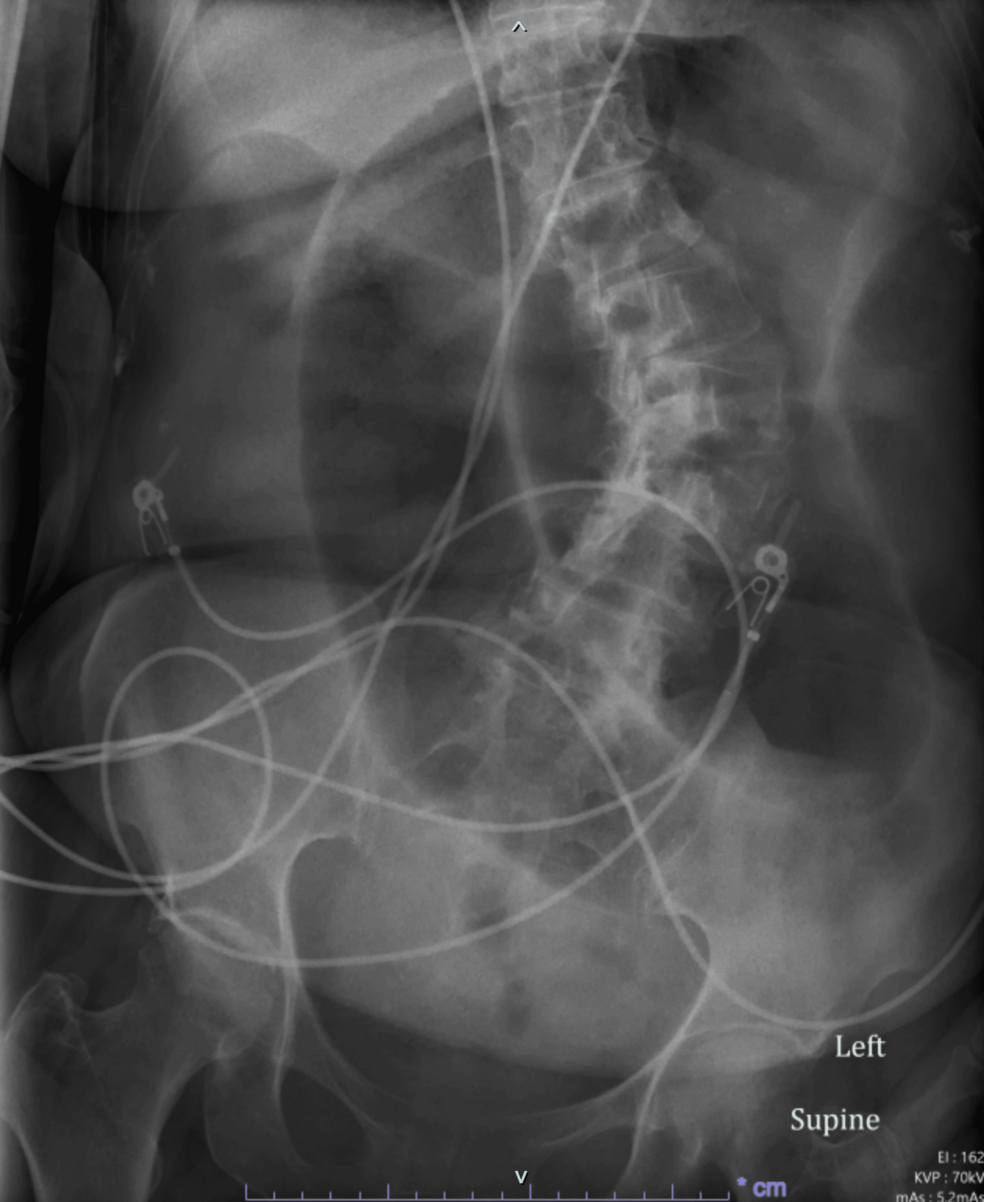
X-ray of the abdomen showing dilated bowel loops, possibly representing a redundant sigmoid colon or sigmoid volvulus.
